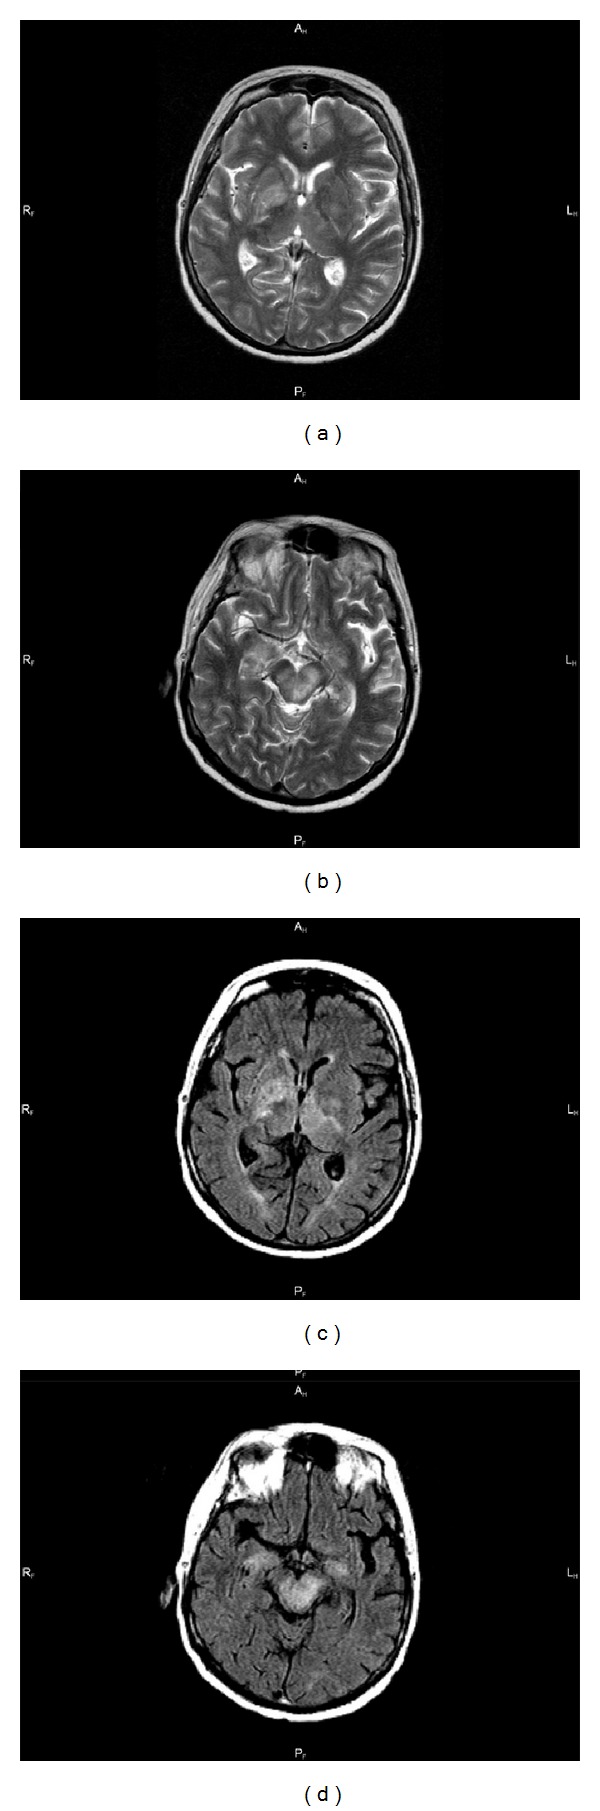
Brain MRI in T2 (a, b) and FLAIR (c, d).
